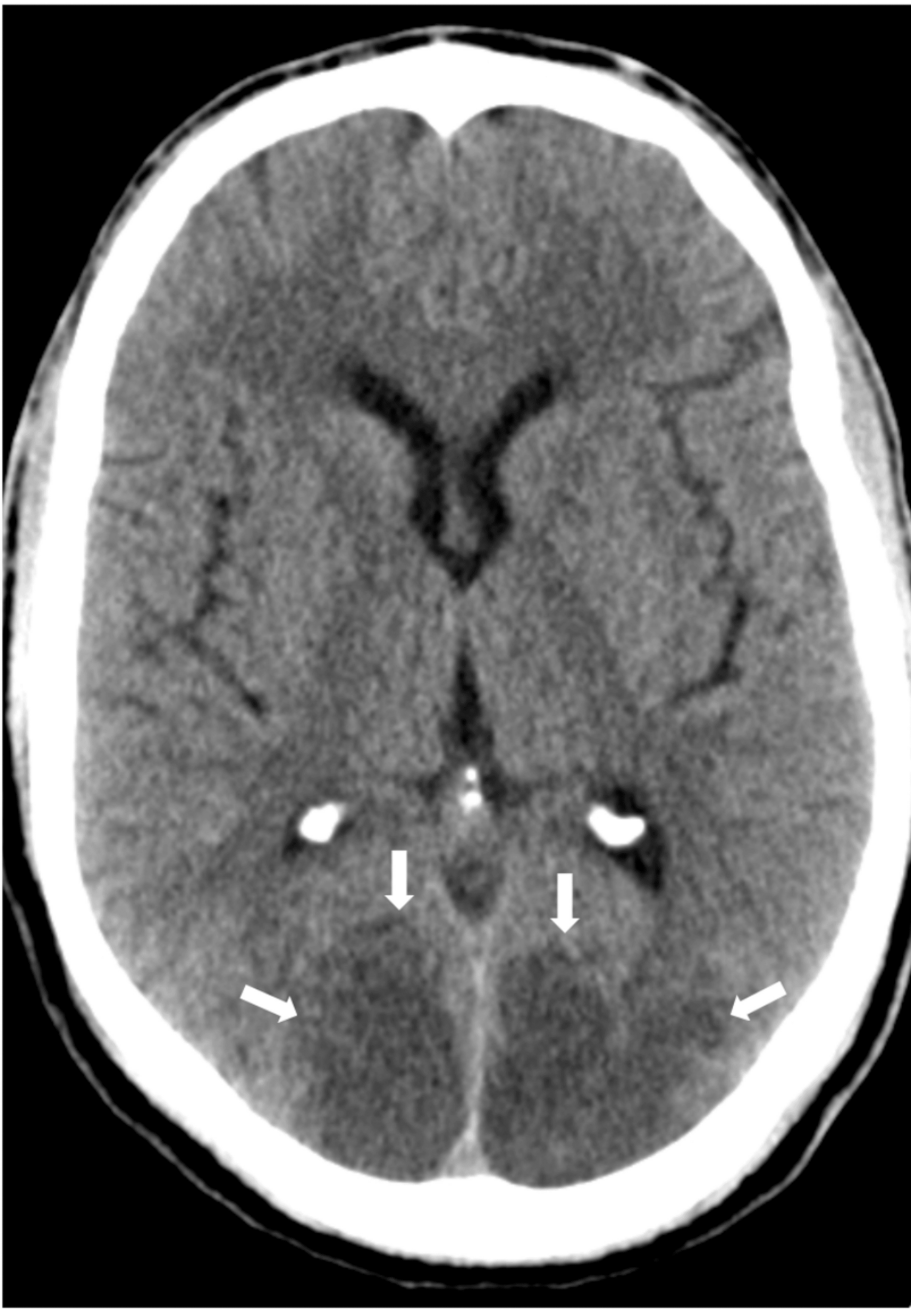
Brain CT scan showing parieto-occipital areas of cortico-subcortical edema CT: computed tomography
